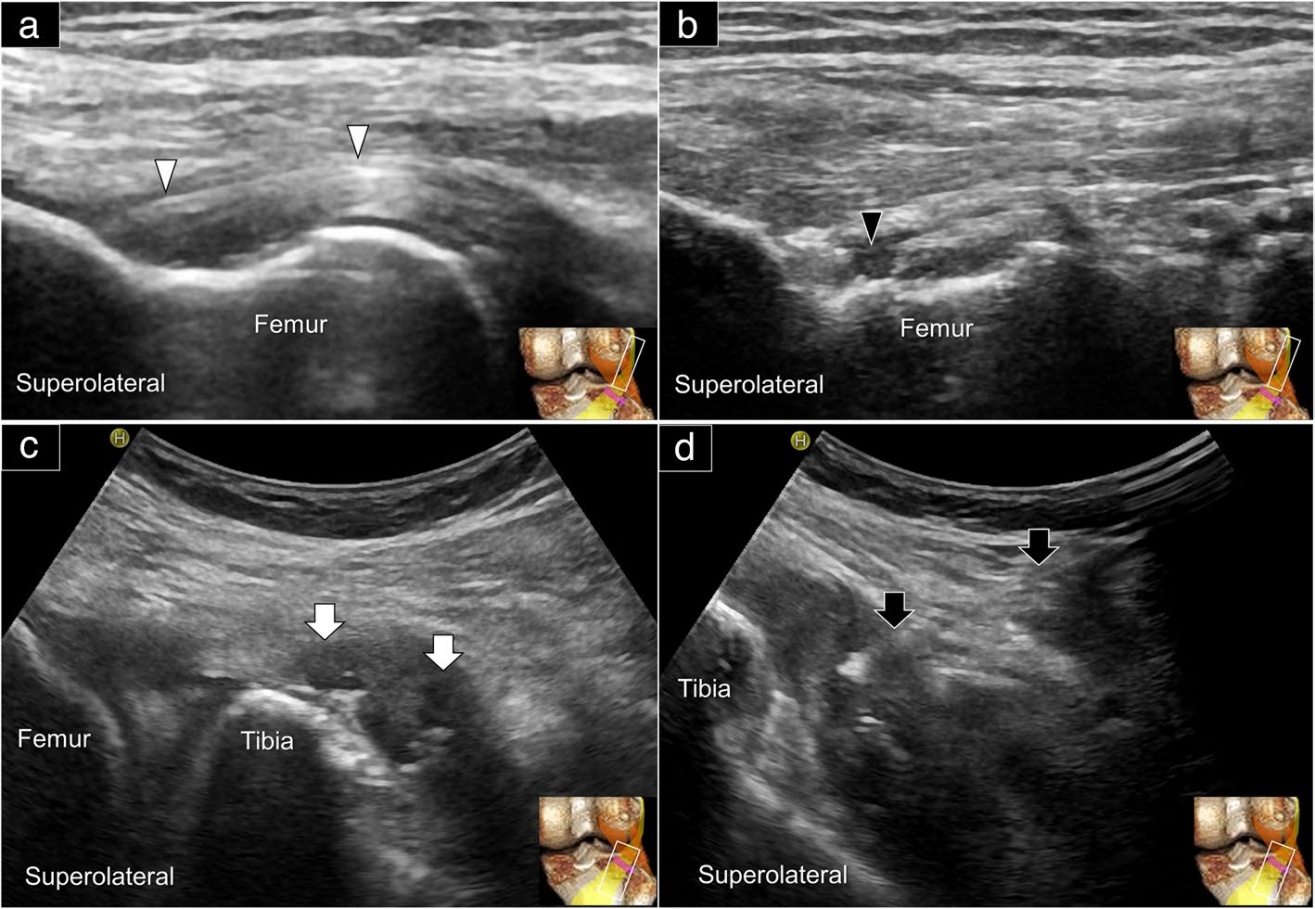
Ultrasound imaging (long-axis view) of the normal (white arrowhead) (a) and partially torn (black arrow) (b) popliteus tendons. Popliteus muscle tear (white arrow) (c) and the ultrasound-guided injection (d). Black arrow: needle trajectory
